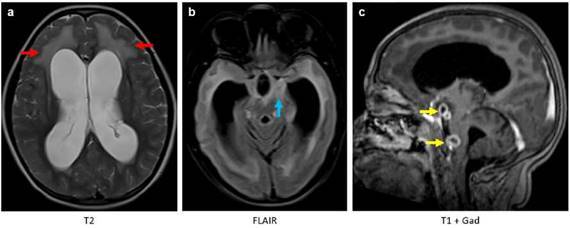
Resonancia magnética cerebral. Imágenes axiales potenciadas en T2 (a), FLAIR (b) y coronales T1 después del uso de gadolinio (c). Hidrocefalia no obstructiva supra e infratentorial con hiperintensidades de la sustancia blanca periventricular (flecha roja) correspondiente a edema transependimario por actividad hidrocefálica. Aumento de la intensidad de señal en las meninges de la base del cráneo (flecha azul) como causa principal de la hidrocefalia por obstrucción del flujo cefalorraquídeo y disminución en su absorción. Se detectaron dos lesiones extraaxiales con realce en anillo, correspondientes a focos de tuberculomas (flechas amarillas).
